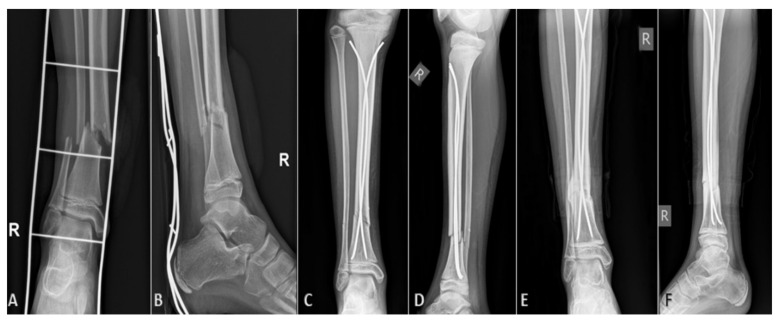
Displaced distal tibial fracture in 9-year-old male patient: (A) preoperative AP radiograph; (B) preoperative LL radiograph; (C) AP radiograph one month after surgery; (D) LL radiograph one month after surgery; (E) AP radiograph three months after surgery; (F) LL radiograph three months after surgery.
